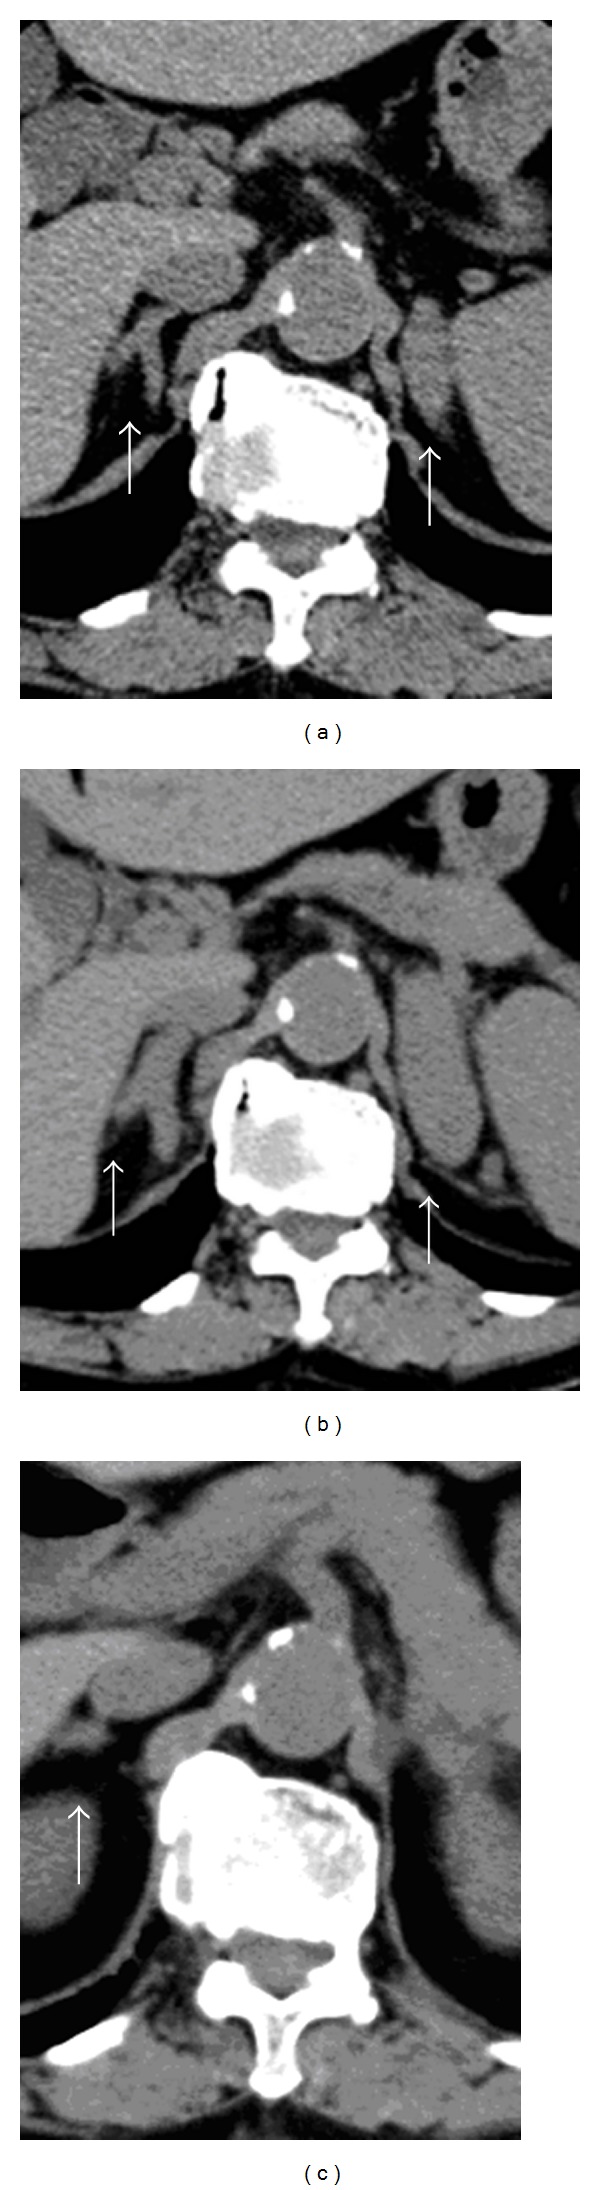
CT image (a) on the first visit; (b) 1 year after the first visit revealing enlarged adrenal glands; and (c) after R-CHOP therapy showing shrinkage of the right adrenal gland.
